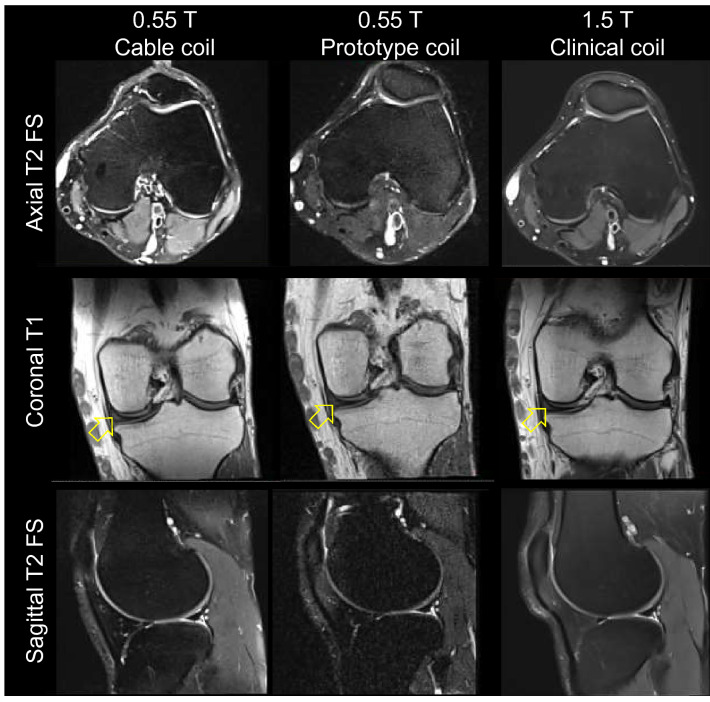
Representative turbo spin echo MR images of a 35-year-old man acquired using the 0.55 T 6-channel cable coil (left column), 0.55 T prototype coil (middle column) and 1.5 T clinical coil (right column). Mucinous degeneration with no meniscal tear is observed in the coronal images (arrows).
